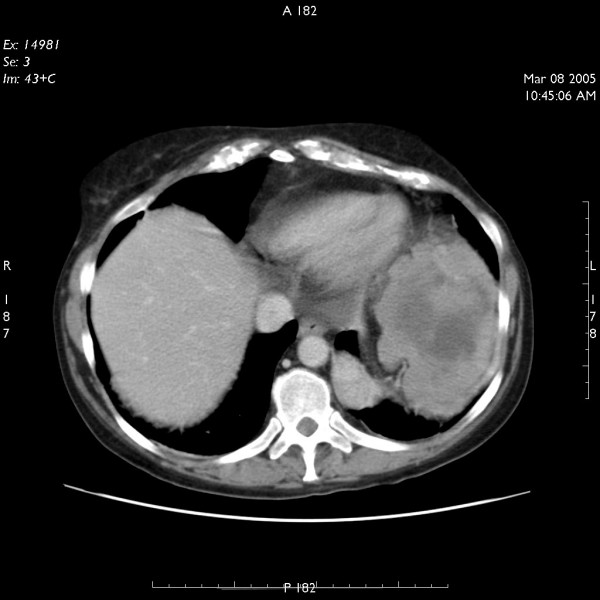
CT scan of abdomen showing extent of primary tumour.
